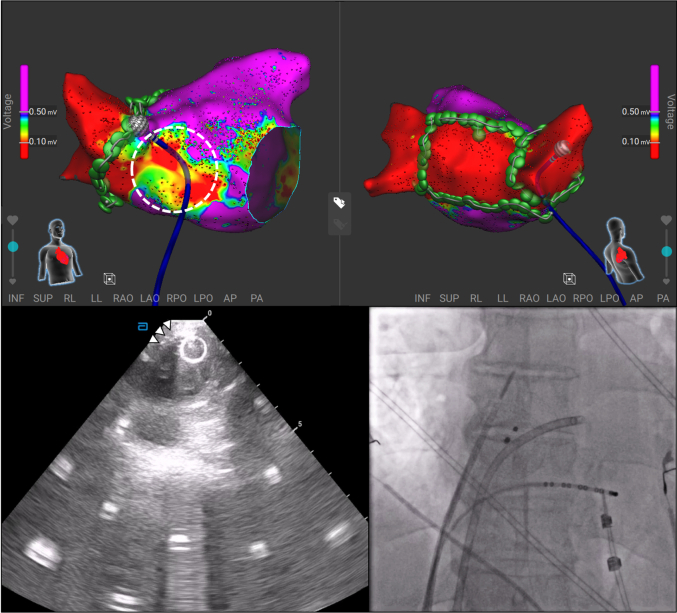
Catheter Position Associated With Temperature Rise and Corresponding Temperature Change (Case 1) During an application near the right superior pulmonary vein, the catheter temperature increased to 61 °C. (Top Left) The white dotted line indicates the estimated location of the occluder. (Bottom Left) On intracardiac echocardiography, the lattice-tip catheter was in proximity to the occluder (arrowheads), and no abnormal bubble stream was observed during the application. (Bottom Right) Left atrial access was successfully achieved by traversing an occluder using a steerable sheath.
